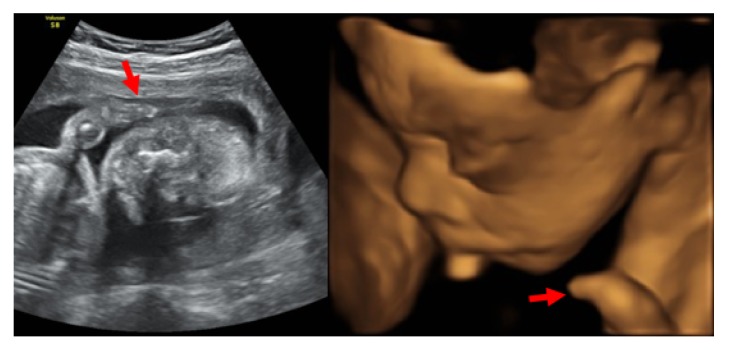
Two-dimensional (right) and three-dimensional (left) prenatal ultrasound images showing short forearms of 7 mm and 9 mm (arrows) and short humeri and femurs (–2.1 standard deviation, SD).
